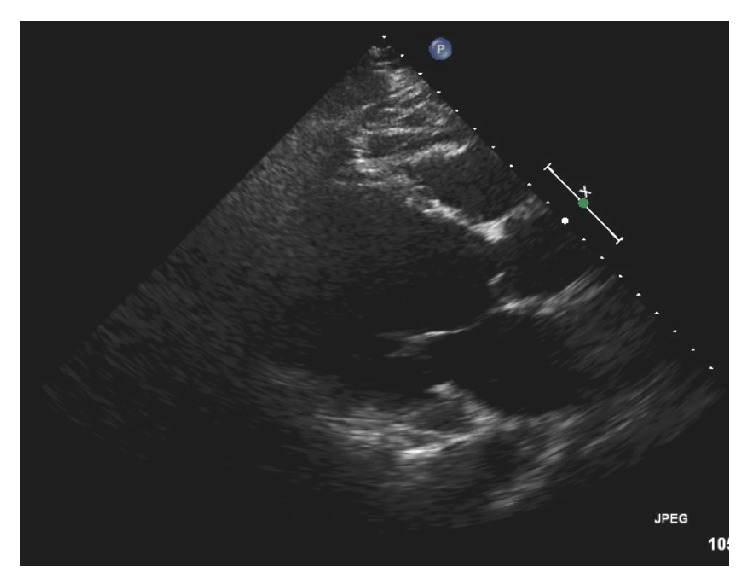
Echocardiogram showing dilated LV and EF of 10%.
